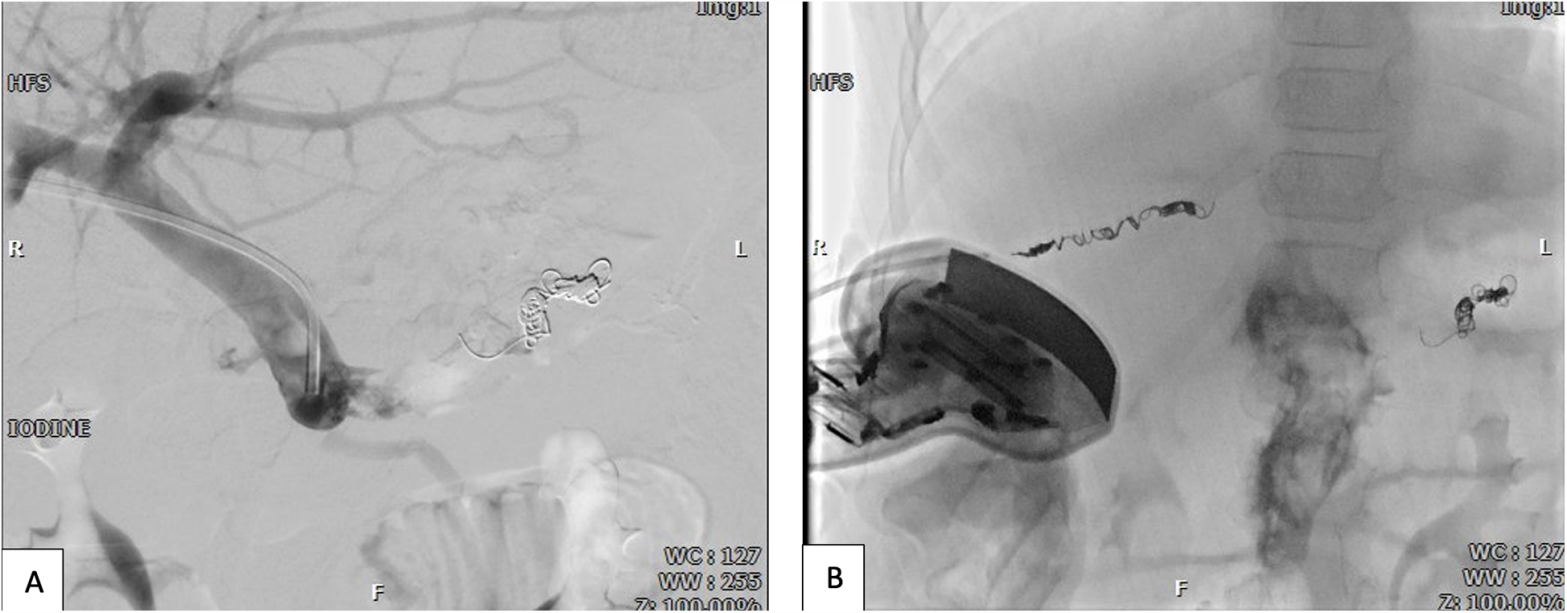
(A) Post-coiling and post-sclerotherapy trans-hepatic portography shows complete obliteration of varicose veins and porto-systemic shunts. (B) Hepatic sheath pathway coiling for hemostasis by guidance of sonography and fluoroscopy.
